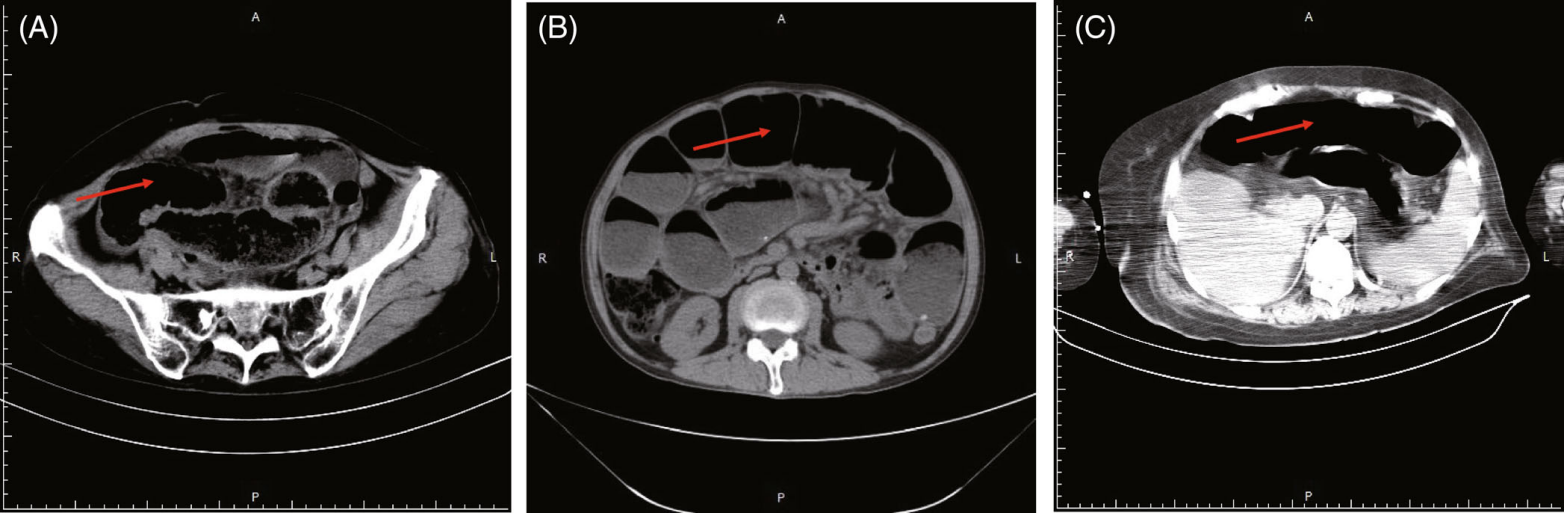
CT of intestinal obstruction prior to perforation. (A) Case 1 images. CT of intestinal obstruction prior to perforation. (B) Case 2 images. CT of intestinal obstruction prior to perforation. (C) Case 3 images. CT of intestinal obstruction prior to perforation.
